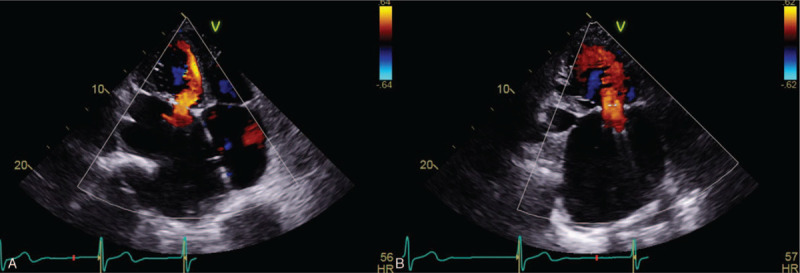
No specific findings were detected in the left atrium other than mitral regurgitation using preoperative transthoracic echocardiography. (A) Apical 4-chamber view. (B) Apical 2-chamber view.
